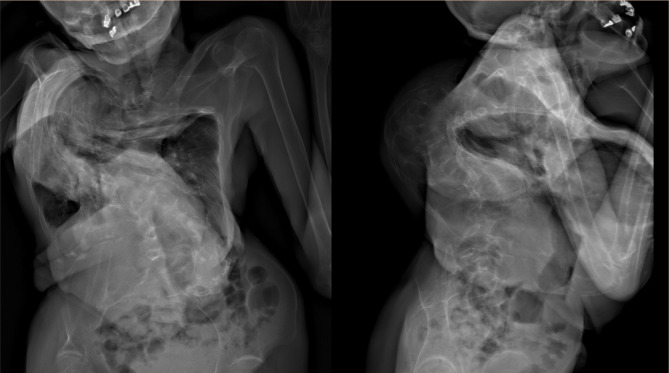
Anteroposterior (left) and lateral (right) full-spine radiographs with a major thoracic curve in a 67-year-old woman. Note the severity of the curve in the coronal plane at Cobb angle 172° and the sagittal plane at 150°.
